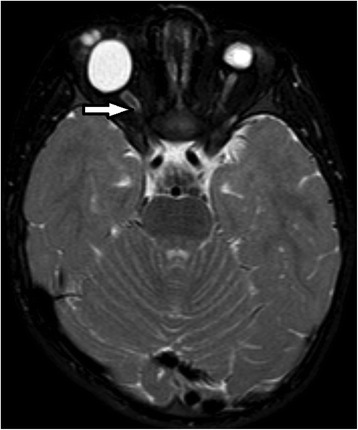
MRI revealed that only the right optic nerve (one *arrow*) was developing
